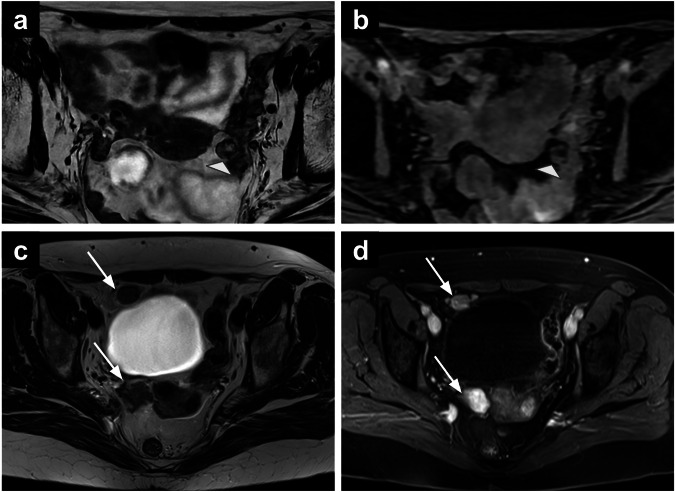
Appendigitis (a, b). Axial T2-WI (a) and fat-suppressed T1-WI (b) in a 48-year-old cervical cancer patient 3 months after CCRT showing epiploic appendagitis (arrowheads). Pelvic splenosis (c, d). Pelvic nodules (arrows in c, d) in a 59-year-old patient with breast cancer who underwent pelvic MRI for characterisation of para-uterine solid lesions detected on a transabdominal ultrasound. These lesions turned out to be sites of pelvic splenosis, related to a previous post-traumatic splenectomy. SI, signal intensity; WI, weighted images
